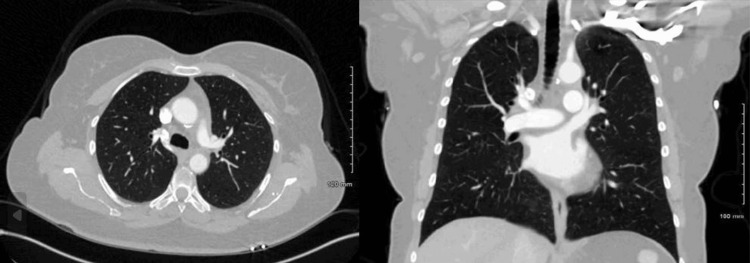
Axial and coronal views of CT chest with contrast approximately one year after resection demonstrating no evidence of disease recurrence
